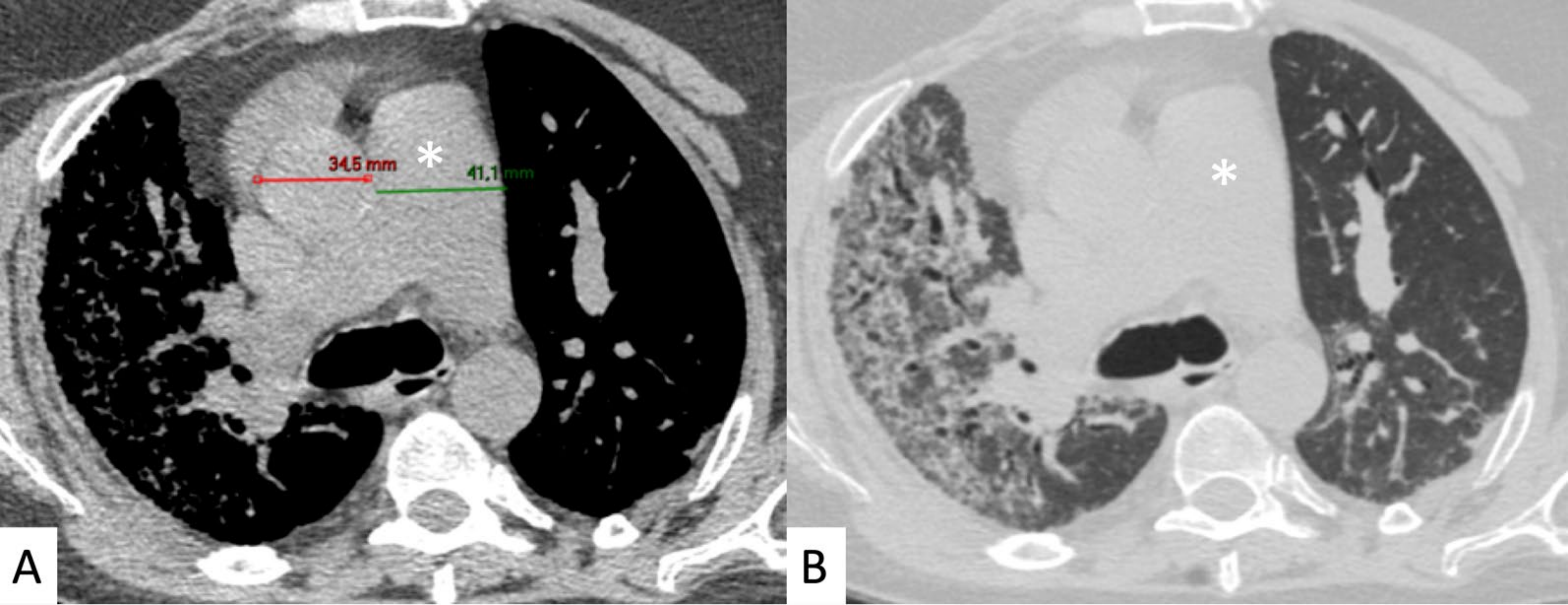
A patient with pulmonary hypertension (PH) assessed by chest CT. A Mediastinal window preset of visualization and (B) lung windowing preset of visualization clearly demonstrate the enlargement of the pulmonary trunk (white asterisk); commonly, the main pulmonary artery/ascending aortic/ratio is calculated. Generally, normal values are less than 1.0, whereas in cases of superior values, PH may be suspected
